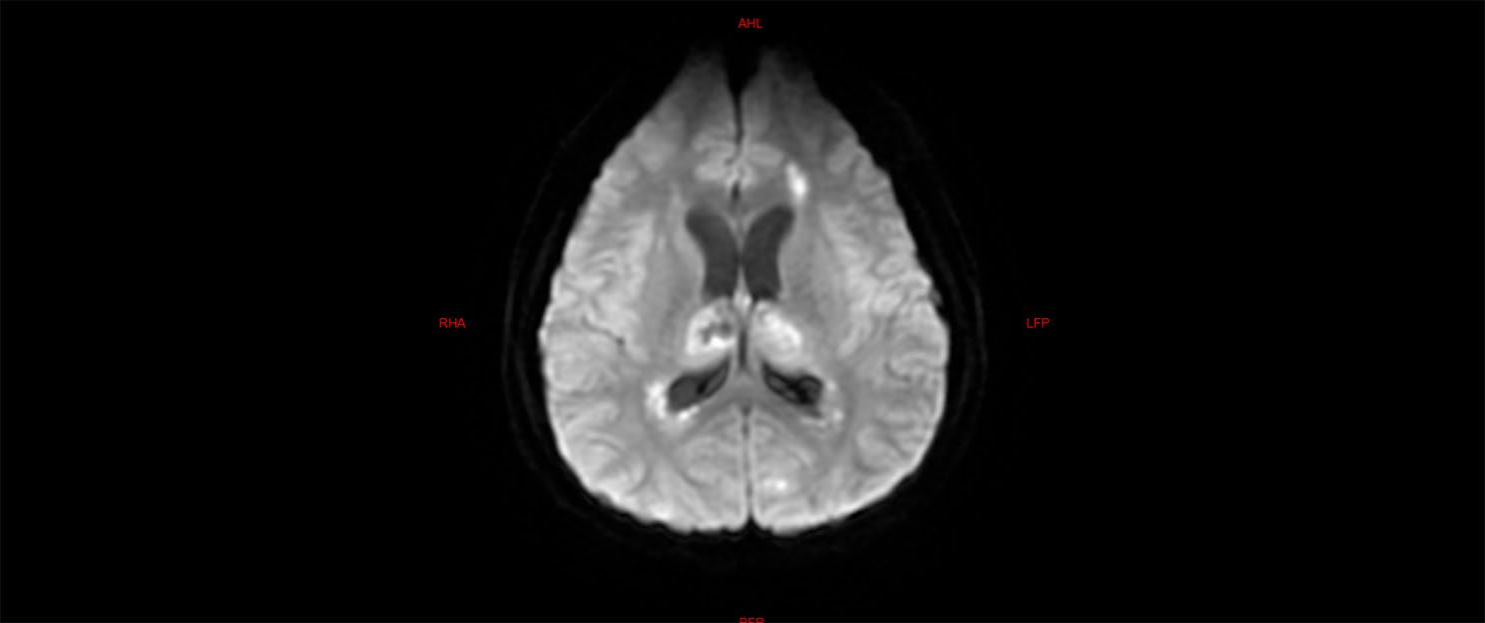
Brain MR DWI sequence of case 6, showed a patchy high signal in the bilateral thalamus and periventricular white matter, and a low signal in the right thalamic lesion, considering bleeding. The patient was diagnosed with necrotizing encephalopathy. Following admission, there were complications of central respiratory failure and circulatory failure, necessitating basic life support through mechanical ventilation and high-dose vasoactive drugs. Despite treatment, there was no improvement, and ultimately, the parents chose to discontinue treatment, leading to the patient’s demise
